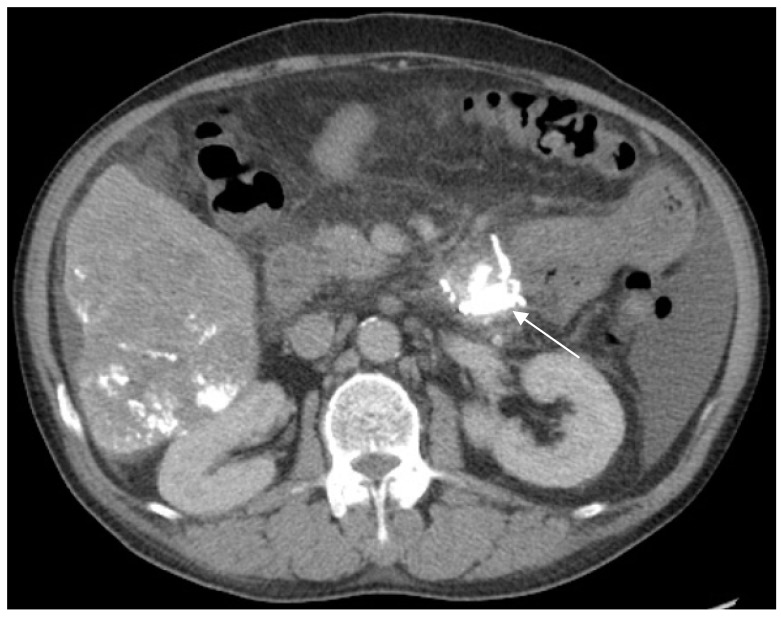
Abdominal contrast-enhanced computed tomography scan confirms the deposition of dense lipiodol in the proximal jejunum four days after sclerotherapy. The previous transcatheter arterial embolization caused some deposition of lipiodol in the liver, and the amount is unchanged from that observed in the prior image.
